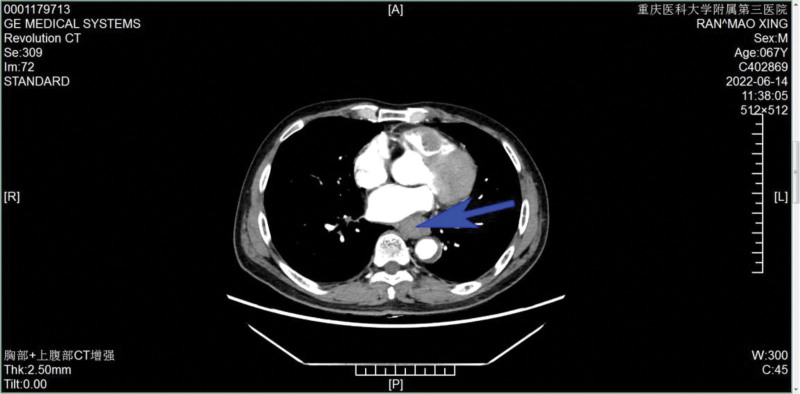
The thoracic computed tomography on June 14th: the location indicated by the blue arrow indicates the possibility of a tumor occupying lesion.
